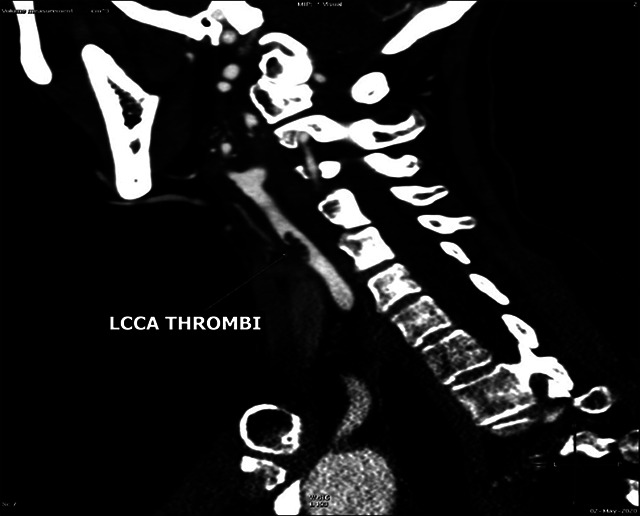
Sagittal reformatted CTA image shows the thrombus floating in the lumen of the left common carotid artery (arrow).
